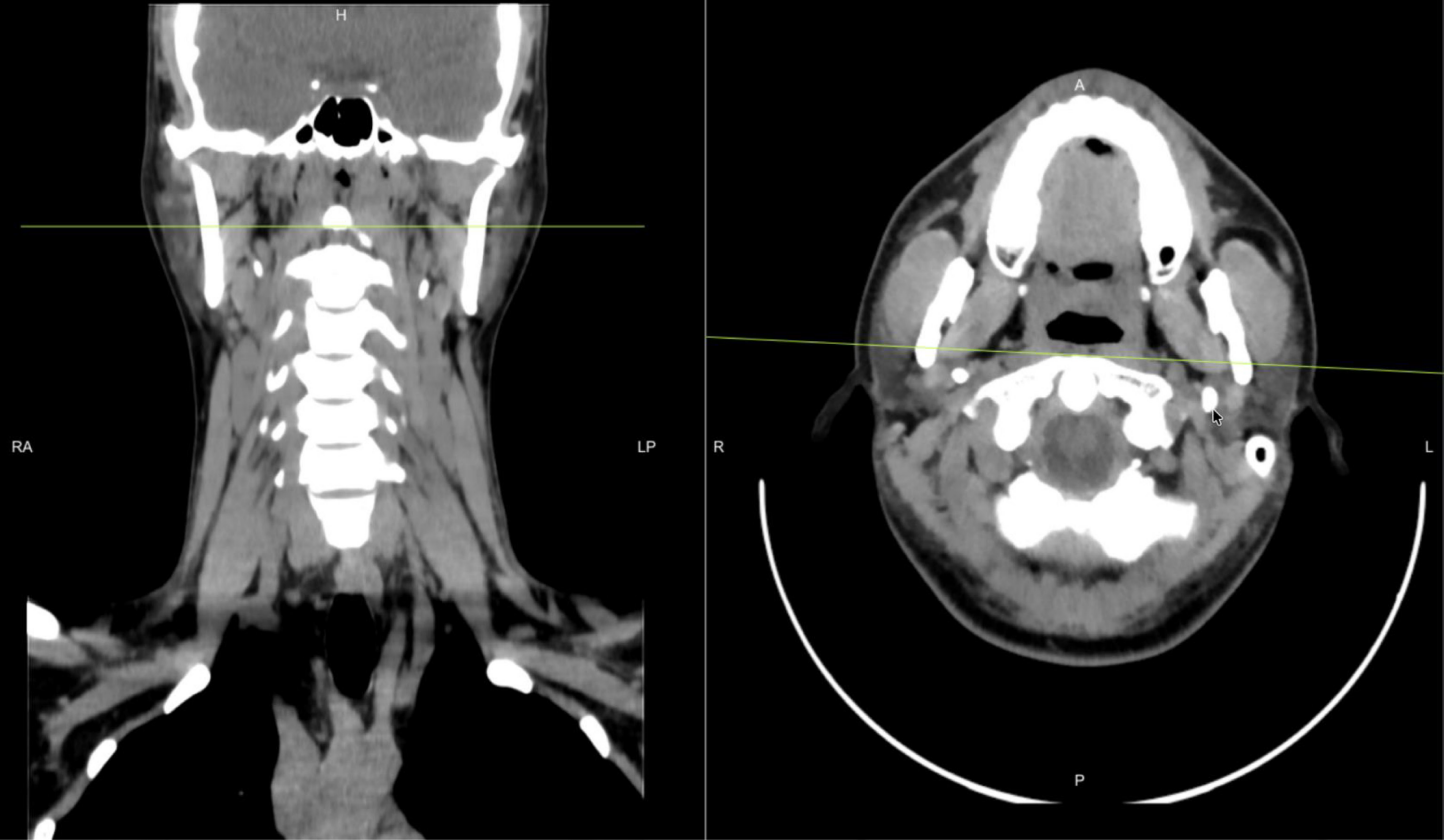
Non–contrast‐enhanced computed tomography (CT) of the neck with axial and coronal reconstructions (bone window) demonstrating bilateral elongated styloid processes.
